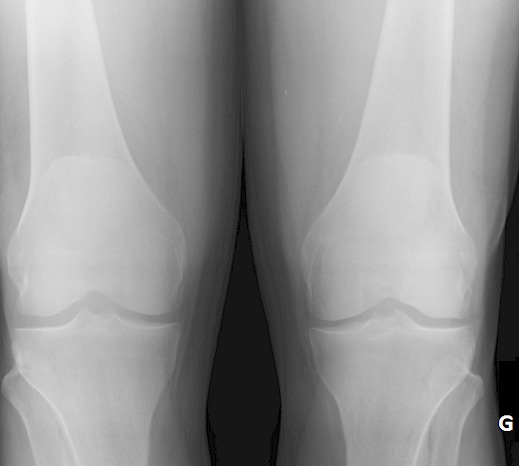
Radiographie de face des deux genoux montrant la position anormalement haute des 2 rotules
